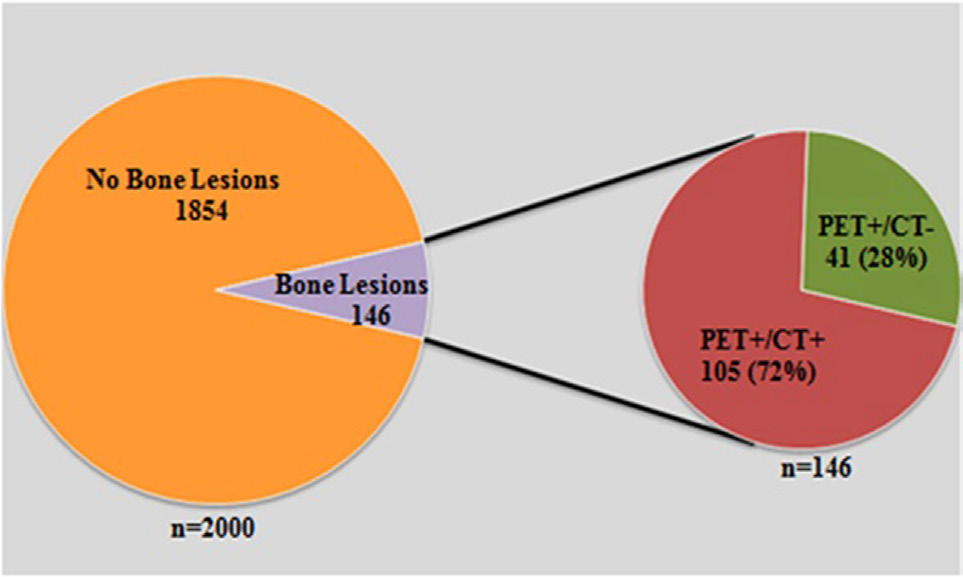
A graph demonstrated total number of scans that were reviewed 2000. Of those, 146 had suspected SM by PET and CT. However, 41 cases were only positive by PET.
